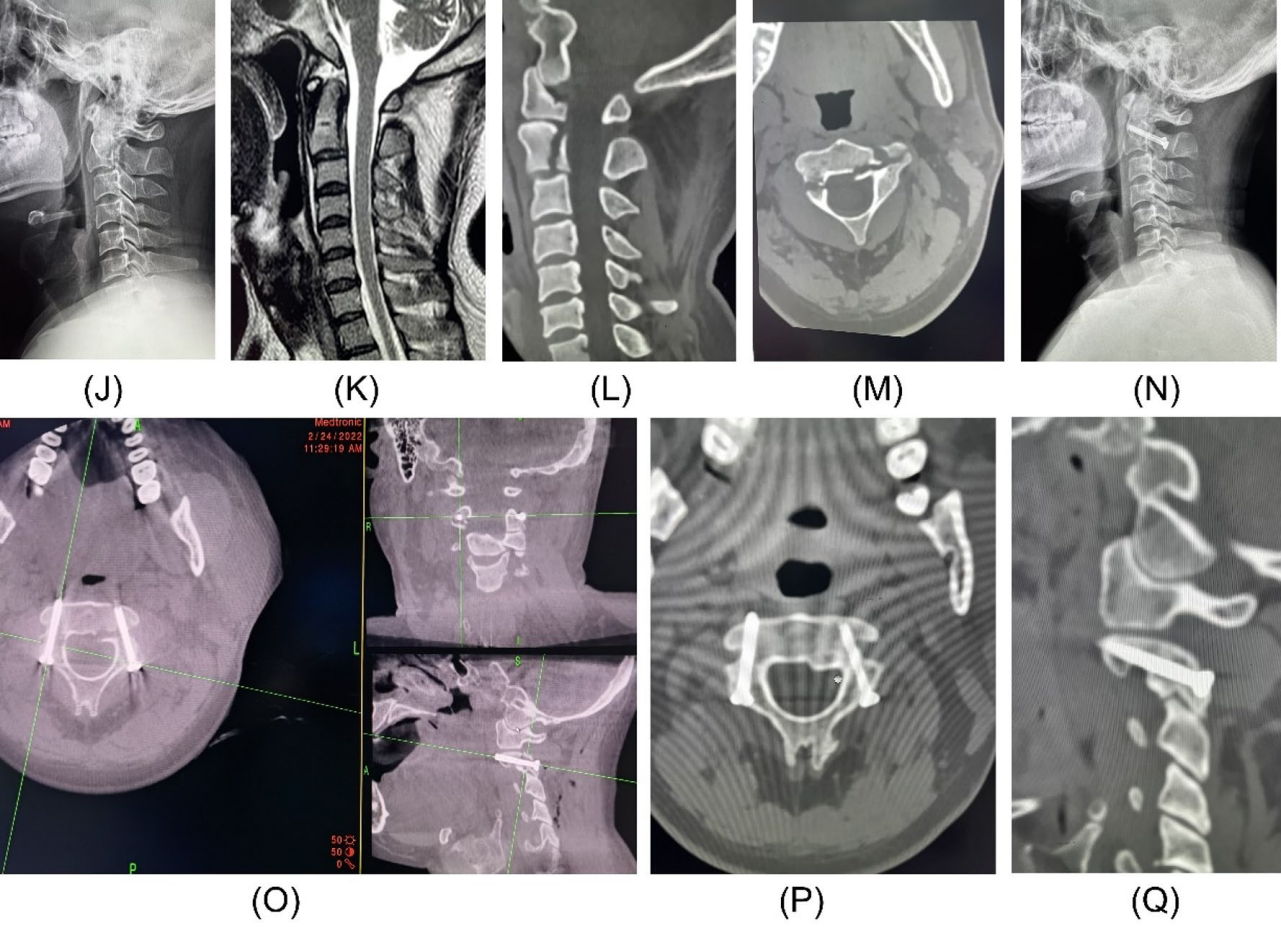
(J) preoperative DR, (K) preoperative MRI, (L–M) postoperative CT, (N) postoperative DR, (P) postoperative CT, (P–Q) last follow-up CT.
